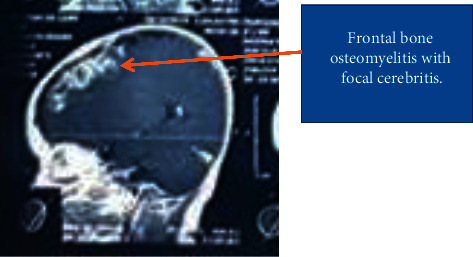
MRI brain of the infant in which left frontal bone osteomyelitis with focal cerebritis can be seen.
